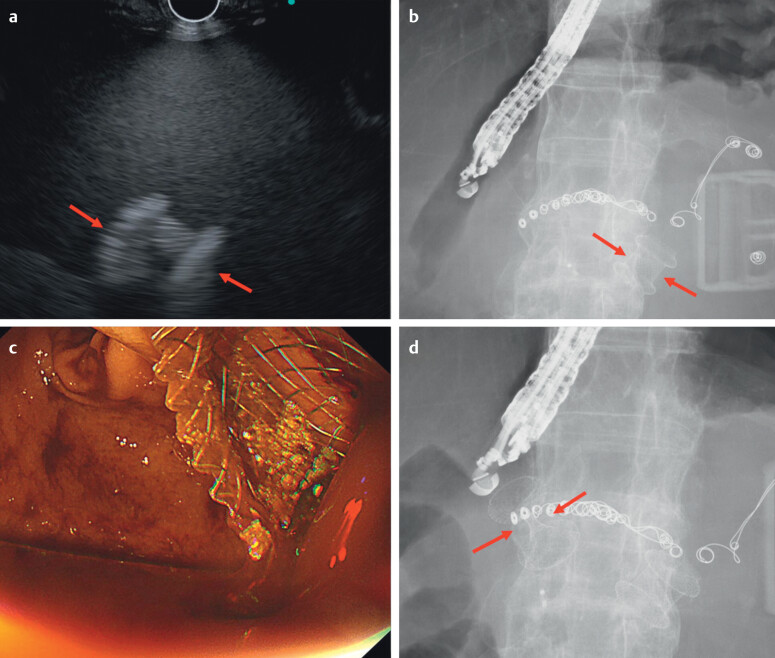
Endoscopic ultrasound (EUS) and fluoroscopic findings.
